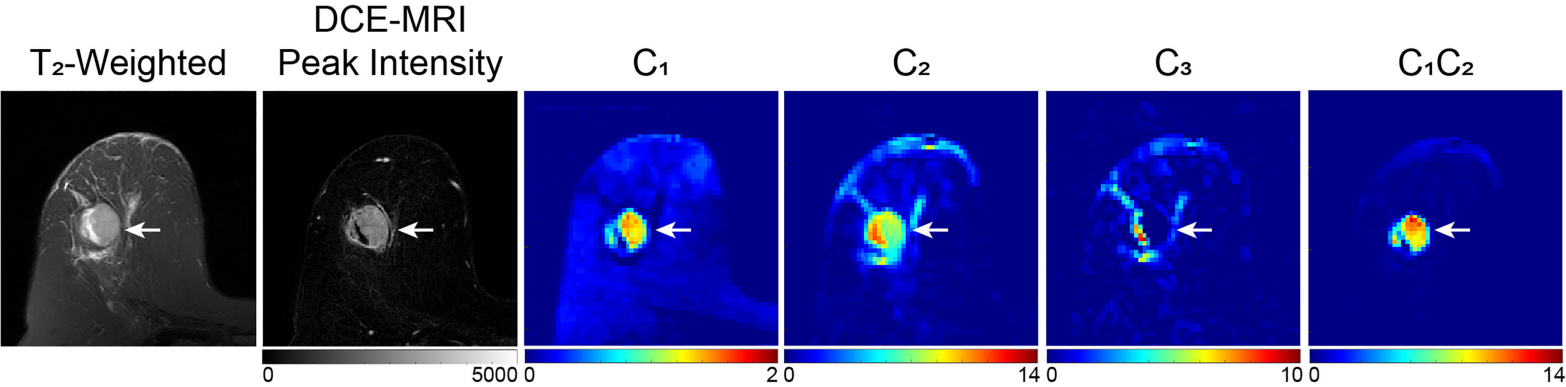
Example of three-compartment RSI analysis in a 49-year-old patient with invasive ductal carcinoma. C_1_, C_2_, and C_3_ maps correspond to the slowest, intermediary, and fastest diffusion compartments, respectively. The lesion, indicated by the white arrow, is hypointense on C_1_ and C_2_ maps compared to surrounding healthy tissue, whereas there is little difference in the C_3_ compartment, which is suggested to correspond to vasculature. The product of C_1_ and C_2_ (C_1_C_2_) results in the greatest tumor conspicuity. DWI images were acquired at b = 0, 500, 1500, and 4000 s/mm^2^ on a 3T scanner, with 50% reduced FOV and without parallel imaging (206).
